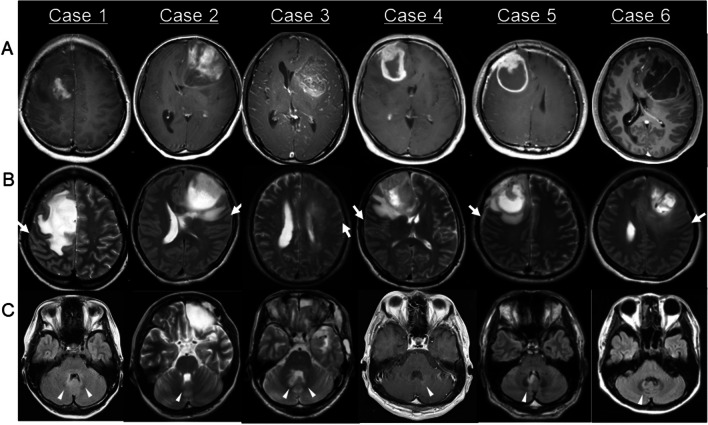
Cases of supratentorial astrocytoma, isocitrate dehydrogenase (*IDH*)-mutant, grade 3 (case 1) and grade 4 (case 2), and glioblastoma, *IDH*-wild type with recurrence in the cerebellar dentate nucleus (DN). A Gadolinium-enhanced T1-weighted magnetic resonance (MR) images (Gd-T1WI) at the initial presentation showing the primary tumor in the frontal lobe in all cases. B T2-weighted MR images (T2WI) at the initial presentation showing the involvement of the hyperintense area in the premotor area in all cases. Arrows indicate the central sulcus. C Fluid-attenuated inversion recovery (FLAIR) (cases 1, 5, and 6) images, T2WI (cases 2 and 3), and Gd-T1WI (case 4) 66, 12, 4, 11, 5, and 5 months after initial tumor resection showing recurrence at the newly developed hyperintense lesions on FLAIR or T2WI or enhanced lesion on Gd-T1WI in the DN and its hilus (arrowheads)
